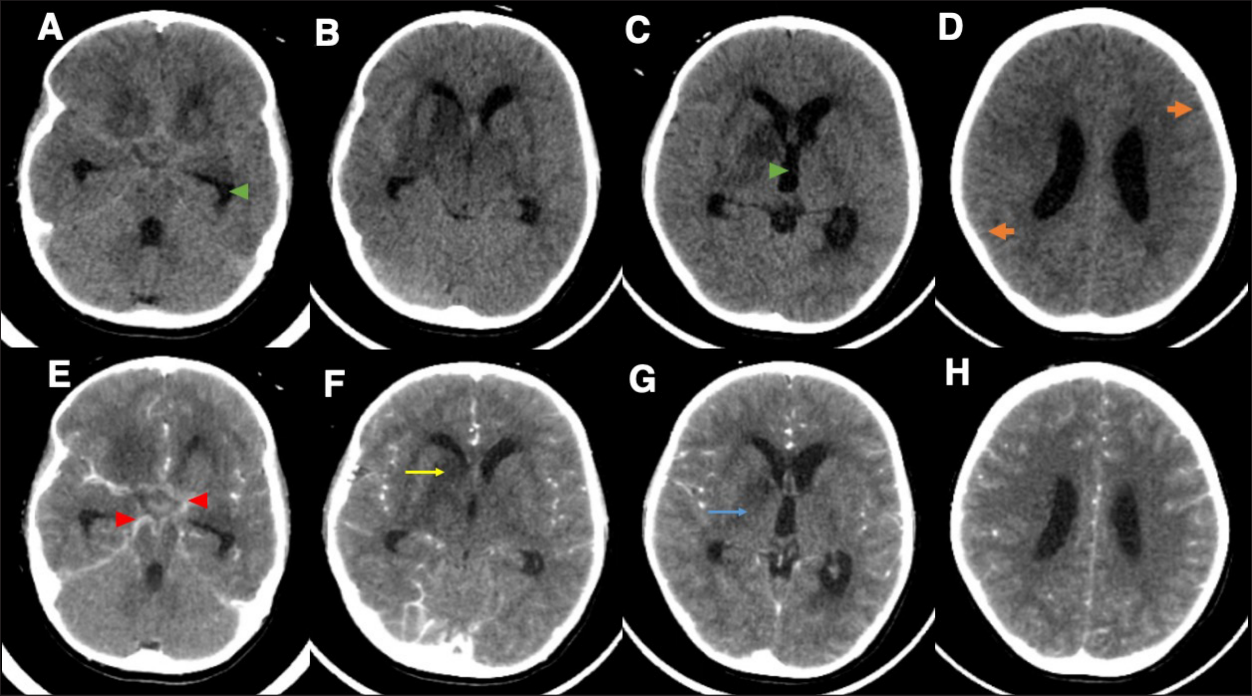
Cranial CT with Contrast. [A-D] Plain cranial CT demonstrating beginning communicating hydrocephalus as evidenced by the dilated temporal horns *(green arrowhead, A)* and third ventricle *(green arrowhead, C)*. Also shown is diffuse cerebral edema as evidenced by the effaced sulci-gyri interface *(thick orange arrows, D)*. [E-H] Contrast-enhanced sequences showing basal enhancements *(red arrowheads, E)* and non-enhancing hypodensities involving the right caudate head *(thin yellow arrow, F)*, right lentiform nucleus, & right internal capsule *(thin blue arrow, G)*. Also seen are undue leptomeningeal enhancements.
